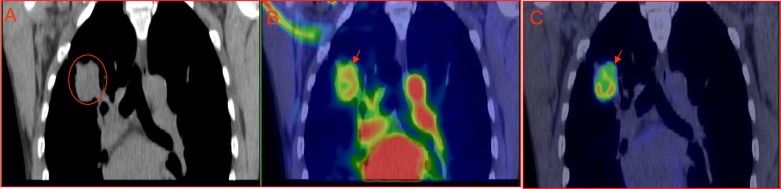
Mismatched intratumoral distribution between early phase perfusion imaging *vs* 60-min metabolism imaging. A 52-yr-old male with pathologically confirmed right lung adenocarcinoma, the patient is given 6 mCi (222MBq) ^18^F-FDG bolus injection and thoracic PET/CT scan starts simultaneously lasting for 5 min (early phase perfusion imaging), the patient has whole body PET/CT scan 60 minutes after injection. There is apparent mismatched between perfusion and metabolism. A: CT imaging; B: perfusion^18^F-FDG PET/CT overlay (0-5min); C: metabolism^18^F-FDG PET/CT imaging.
